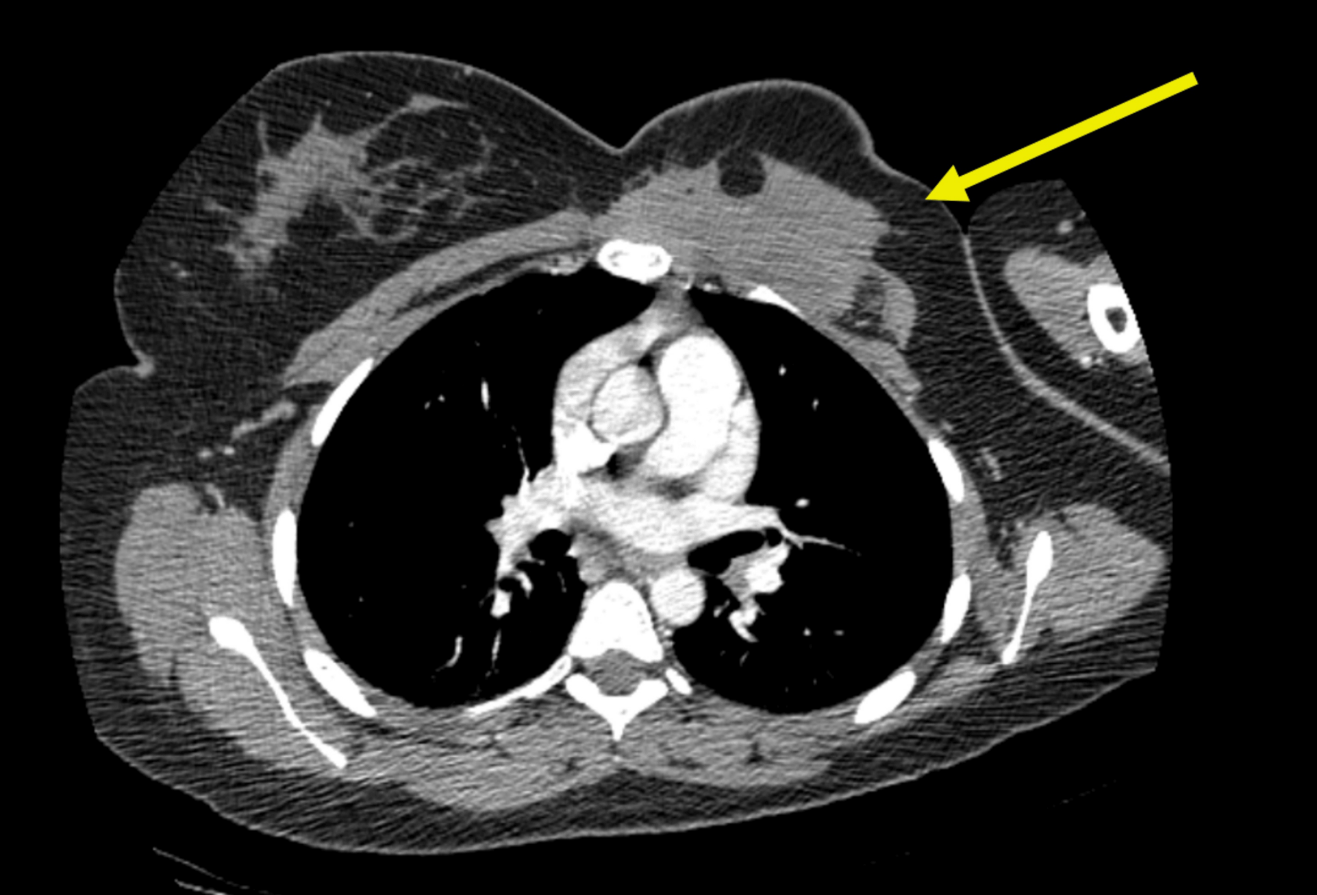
CT Thorax with contrast in the transverse plane which demonstrates a partially enhancing spiculated mass in the left anterior chest wall with associated skin thickening and irregularity.
